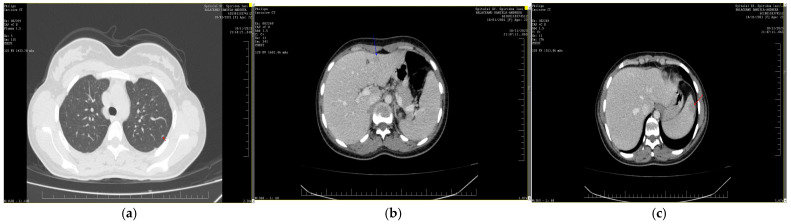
CT scans from thorax (a), liver (b), and spleen (c) showing multiple hypoechoic, circumscribed, randomly distributed nodules situated in both lungs, liver, and spleen and enlarged lymph nodes.
